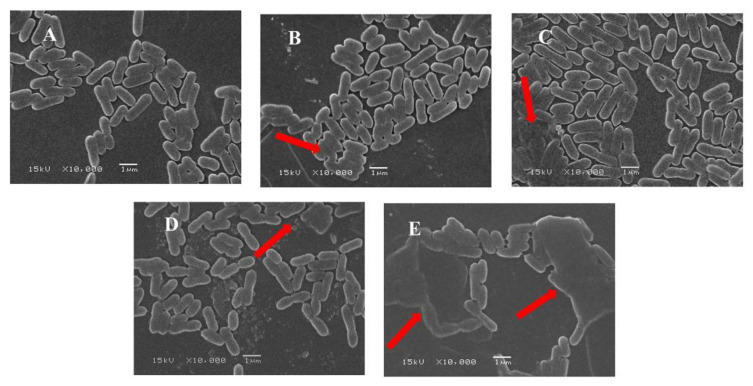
SEM images of *Listeria monocytogenes.* (A) Control group without treatment; (B) Curcumin-treated group; (C) Light-illuminated group; (D) Ultrasound-treated group; (E) SPDT: Curcumin-mediated sono-photodynamic treated group.
